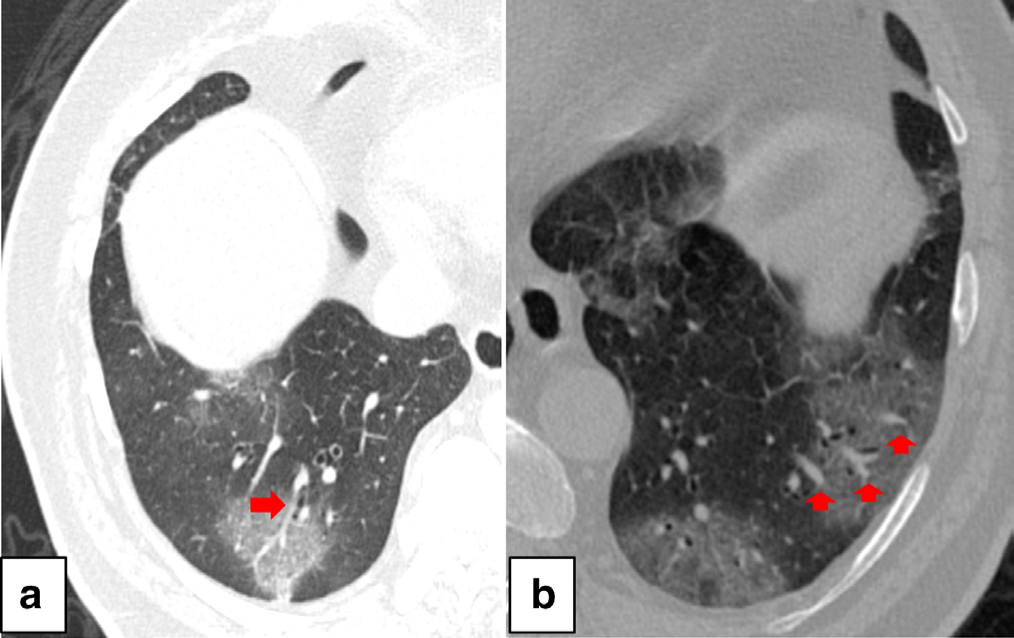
Axial chest CT images in lung window settings obtained through the lower
